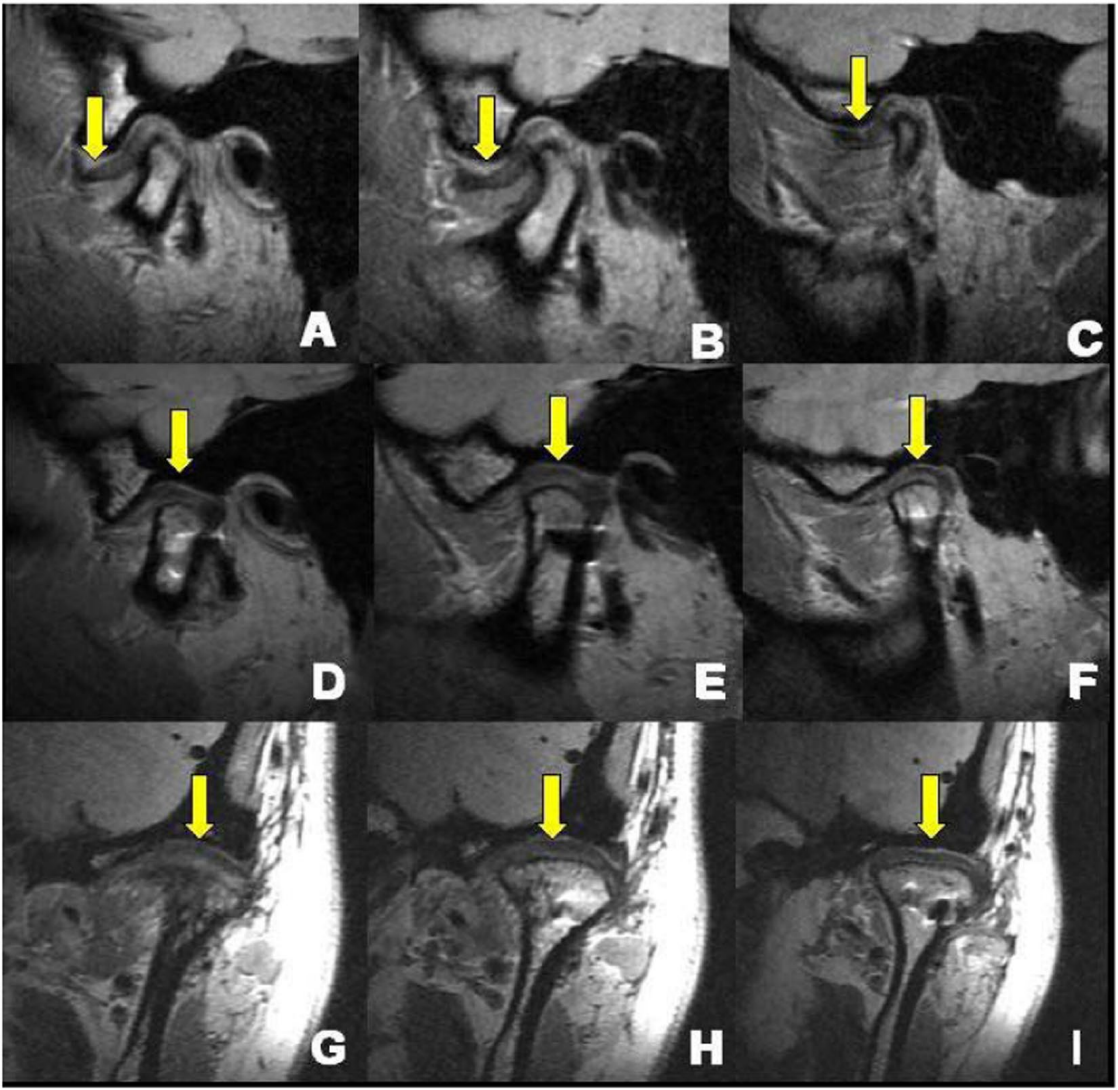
Disc position visualised by MRI before and after surgery – example of A classification. (A–C) show the displaced disc anterior to the condyle (yellow arrow). (D–I) show the displaced disc repositioned to the normal position in two different directions (yellow arrow). Based on the imaging findings this case was classified as (A).
